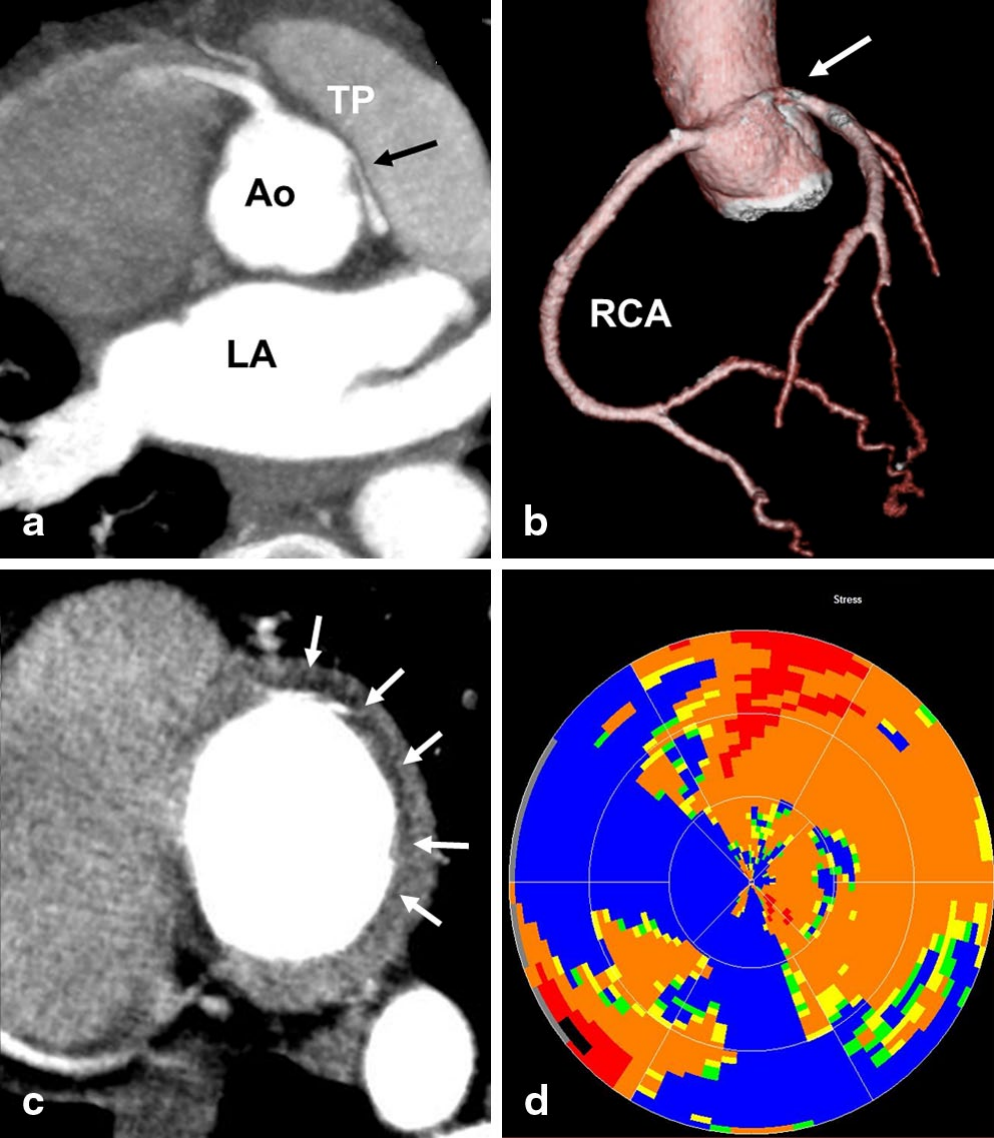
Computed tomography coronary angiography and myocardial perfusion imaging
